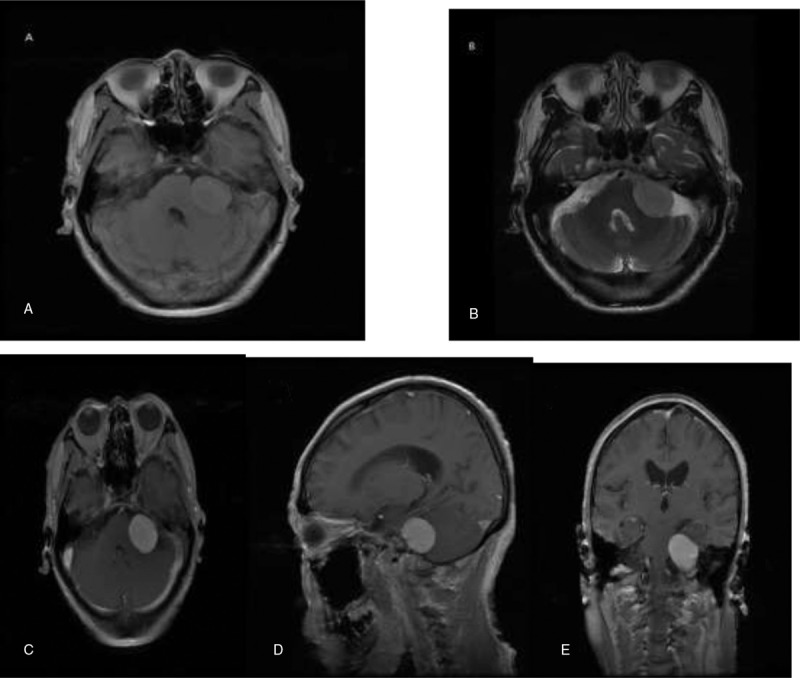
The brain MRI demonstrated a 2.4 × 2.5 × 2.6 cm ovoid mass in the left CPA. The tumor showed intermediate-slightly increased signal intensity in T1WI (A) and slightly increased signal intensity in T2WI (B). Axial (C), sagittal (D), and coronal (E) enhanced T1WI showed homogeneous and strong enhancing tumor, extending into the enlarged IAC and closely connected to the vestibulocochlear nerve. CPA = cerebellopontine angle, IAC = internal auditory canal, MRI = magnetic resonance imaging.
